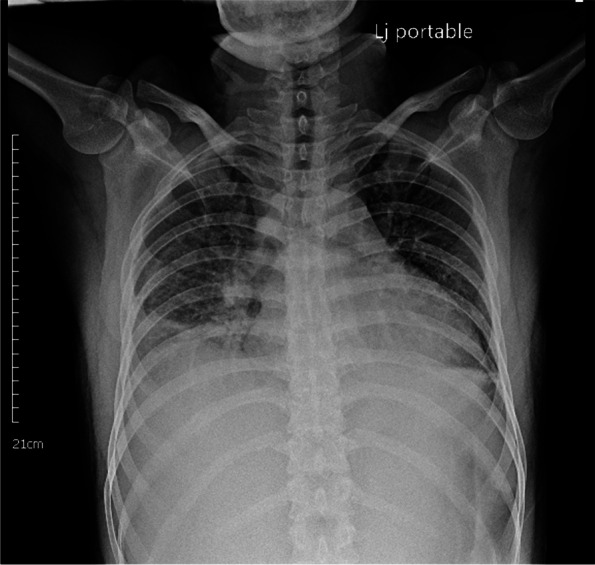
A chest radiography revealed bilateral blunt costodiaphragmatic angle and cardiomegaly
